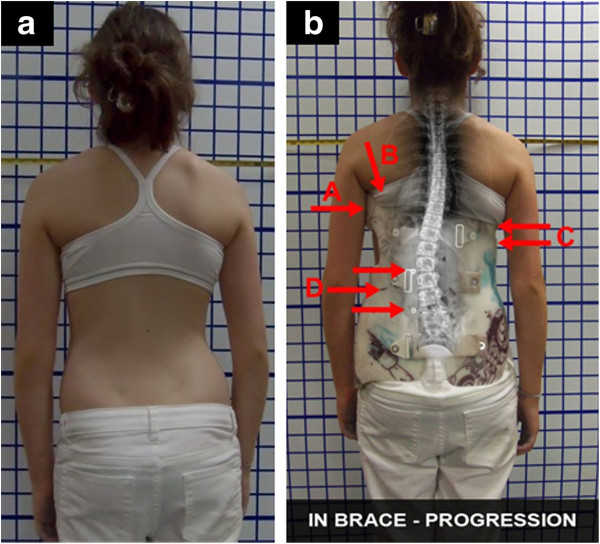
TLSO-type brace with low axilla, thoracic and lumbar pads which causes scoliosis progression. a: The clinical presentation of the patient with a right thoracic curve of 22-degree Cobb angle and a left lumbar curve of 22-degree Cobb angle. b: The in-brace X-ray showed a scoliosis which was worse in-brace compared to out-of-brace; TLSO-type in-brace was thoracic 22-Cobb and lumbar 25-degree Cobb angles. This was the direct result of firstly, the axilla extension being too low (A), which caused the buckling effect (B), and secondly, the low thoracic pad (C) blocking the lumbar correction (D). Furthermore, it was causing lumbar curve progression in the brace.
